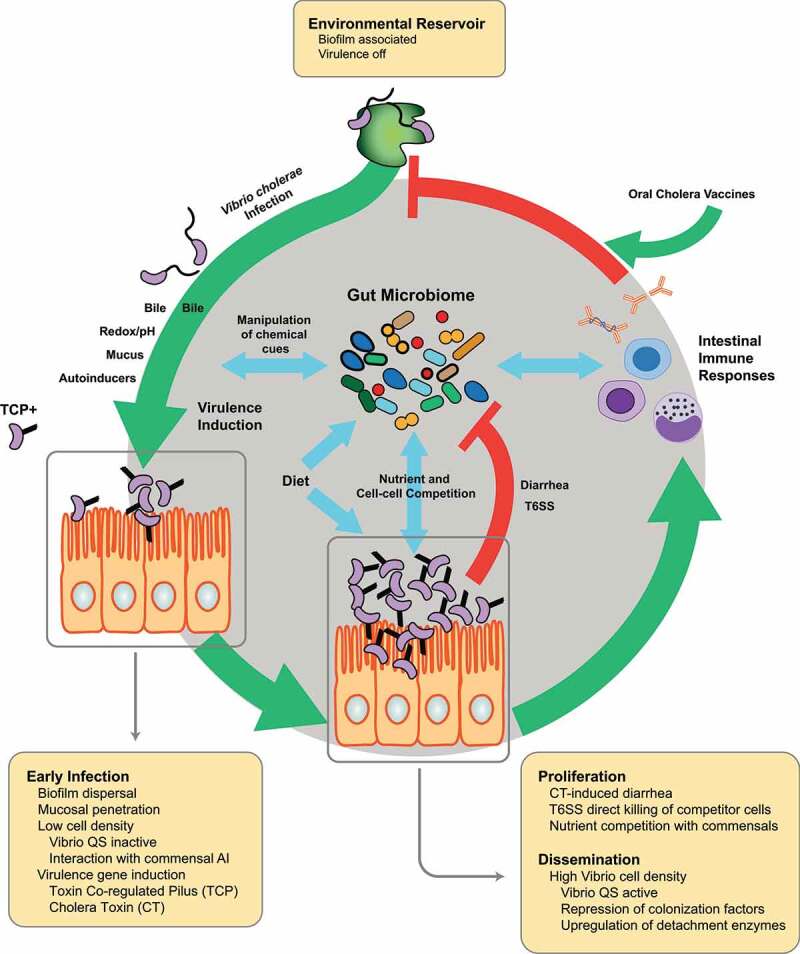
The life and infection cycle of *V. cholerae* and its interaction with the gut microbiome. The gut microbiome interacts with multiple aspects of *V. cholerae* pathogenesis. *V. cholerae* persists in environmental reservoirs, often in the form of biofilms that promote environmental persistence and protection from host gastric acid barriers on infection. The transition into human communities leads to coordinated changes in gene expression modulated by environmental factors such as bile, redox, pH, mucus, and quorum sensing, which are all influenced by the metabolism of gut commensals. This process regulates the expression of key virulence factors such as cholera toxin (CT) and the toxin coregulated pilus (TCP) that are required for diarrhea and colonization respectively. In early infection, *V. cholerae* disperse from biofilms. At low *V. cholerae* cell density in this phase, quorum sensing is inactive and virulence gene expression is active, subject to interaction with commensal-derived autoinducer molecules that can subvert this regulatory process. As *V. cholerae* proliferates, environmental changes in the gut promoted by diarrhea and other factors, alongside type VI secretion activity (T6SS) and nutrient competition, contributes to the clearance of competitor microbes. During late infection, the density of *V. cholerae* cells and quorum sensing molecules is high, leading to repression of virulence and upregulation of factors associated with detachment from the intestinal mucosa and dissemination into the environment. Host immunity, whether from previous natural infection or oral cholera vaccination, can modulate the susceptibility of subsequent hosts to infection from disseminated *V. cholerae*. The diversity of metabolic functions encoded by the gut microbiome determines several aspects of this cycle, including the timing of coordinated gene expression in different phases of infection, competition and persistence *in vivo*, and immune response. The interpersonal variation of the microbiome and the biochemical functions of the commensal microbial community of the gut may thus serve as potent determinant of *V. cholerae* behavior in human populations
